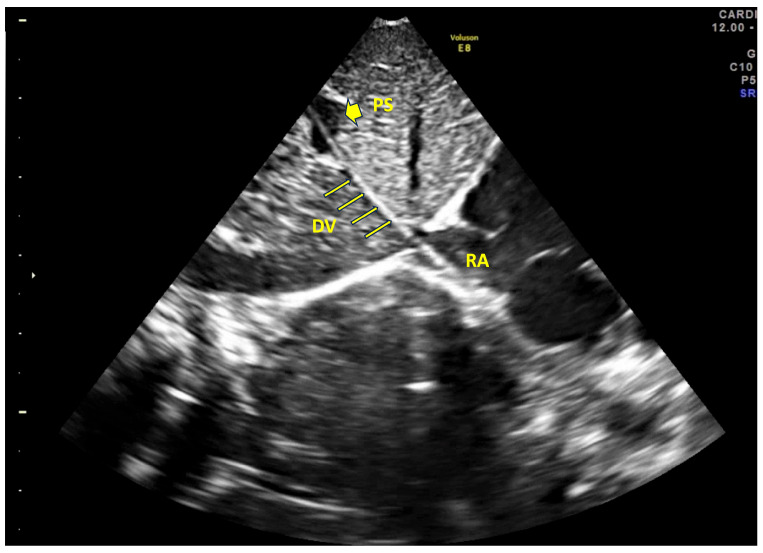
Ultrasound image. In this acoustic window, the portal sinus (PS), ductus venosus (DV), and right atrium (RA) are clearly aligned, facilitating the correct advancement of the catheter. The catheter is visible along its course, with the tip located in the right atrium.
